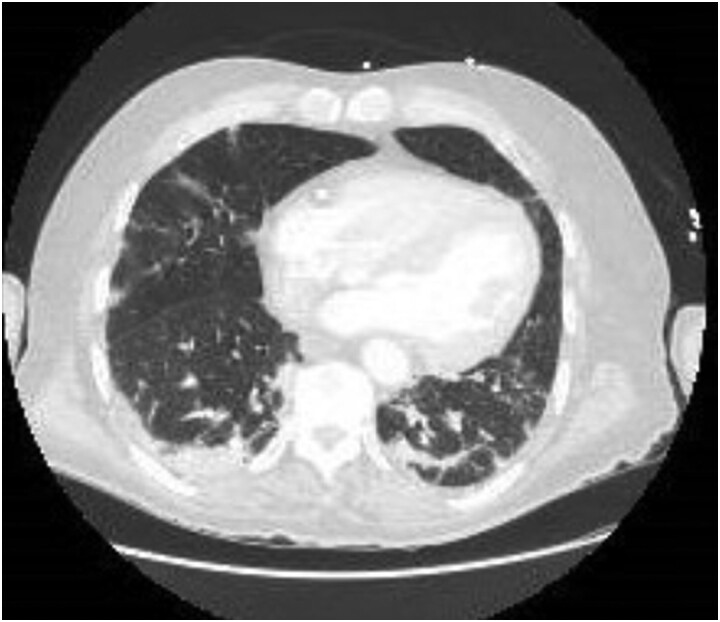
Computed tomography angiogram consistent with COVID pneumonia.
